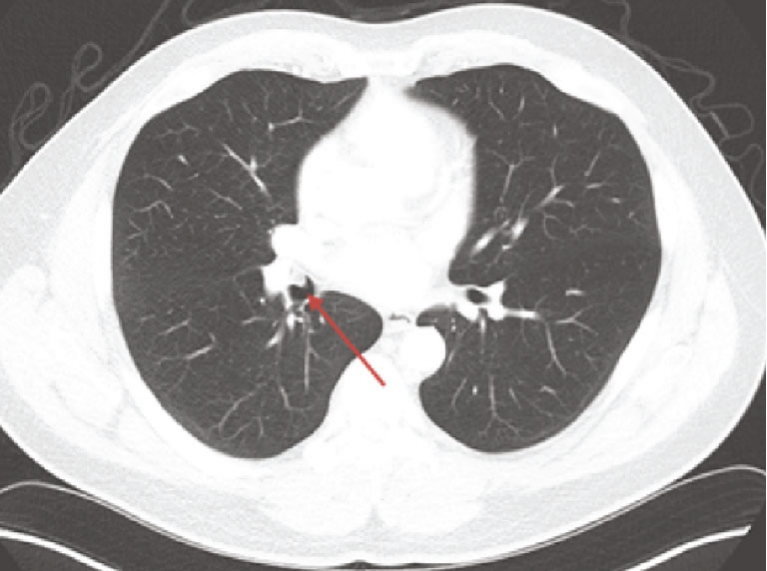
CT chest, axial view: red arrow denoting a 15 mm intraluminal, lobulated exophytic soft lesion at the origin of the right middle lobe.
